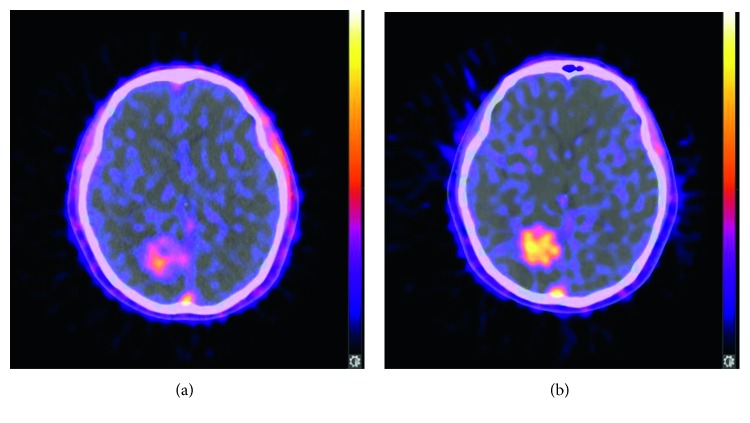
Pre-CK PET/CT image (a) and post-CK PET/CT image (b) of a female patient, 61 years old, diagnosed with lung cancer brain metastases, showing an obvious and uniform increase of [18F]ML-10 uptake.
